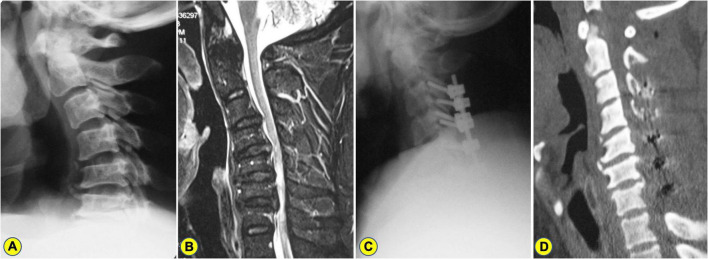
A 51-years-old man who underwent laminectomy with lateral mass screw fixation. (A) The preoperative lateral X-ray shows the C2-7 Cobb angle and curvature index (CI) are 17^°^ and 9.45%, respectively. (B) Preoperative magnetic resonance imaging (MRI) showing C3–7 spinal cord compression combined with kyphosis. (C) A postoperative lateral X-ray showing laminectomy with lateral mass screw fixation. (D) On the CT image of 3 years after surgery, the C2-7 Cobb angle and CI were 6^°^ and 13.09%, respectively.
